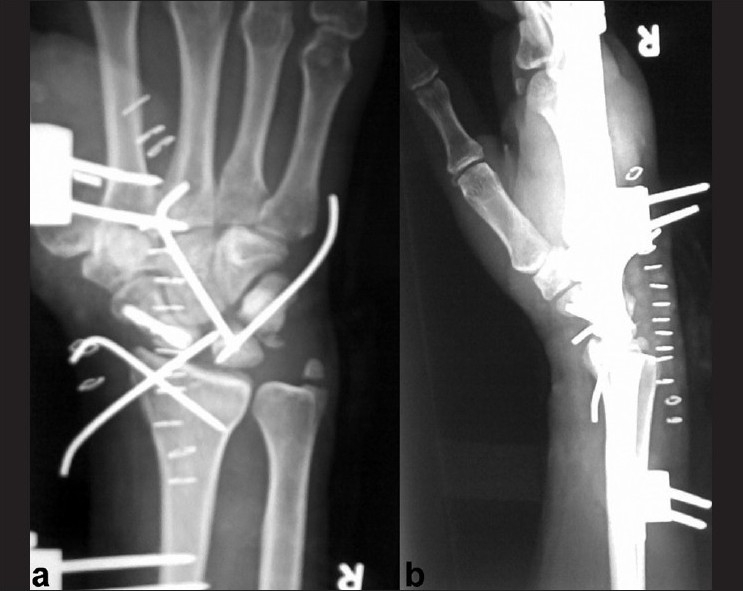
Postoperative anteroposterior (a) and lateral (b) radiograph showing good carpal alignment
